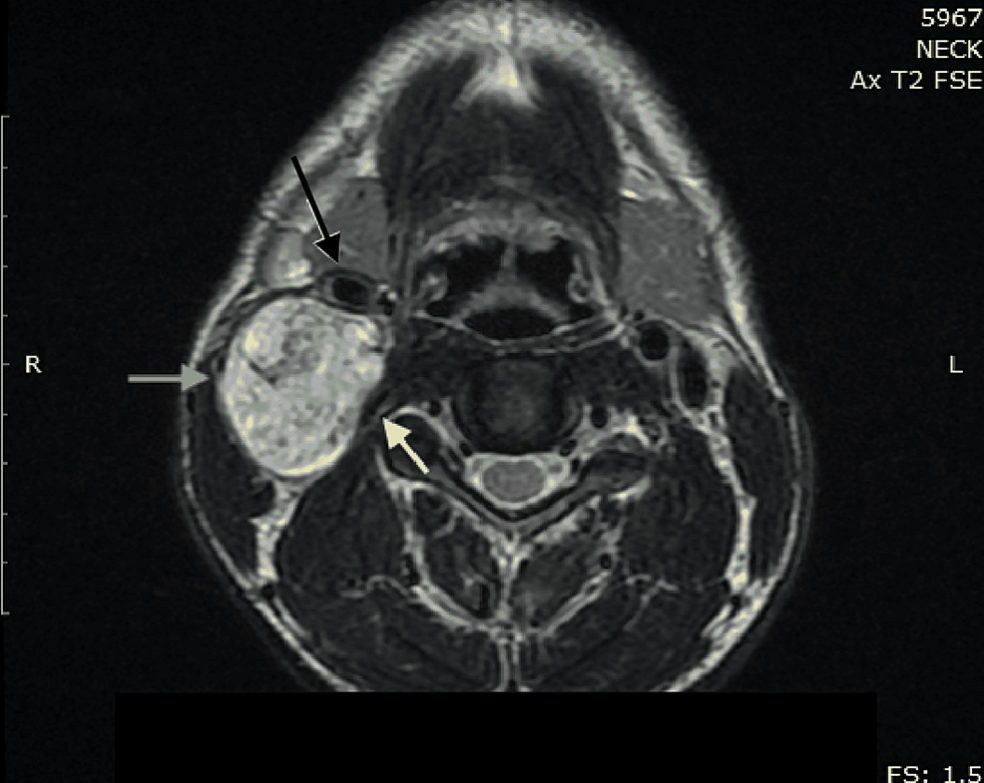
MRI of the neck Axial view. Gray arrow: well-circumscribed, highly-vascularized mass with dimensions of 3.5 x 3.5 x 1.6 cm in the right lateral cervical region directly under the right sternocleidomastoid muscle, separating the internal jugular vein and the carotid artery. Yellow arrow: compressed internal jugular vein. Black arrow: carotid artery MRI: magnetic resonance imaging
